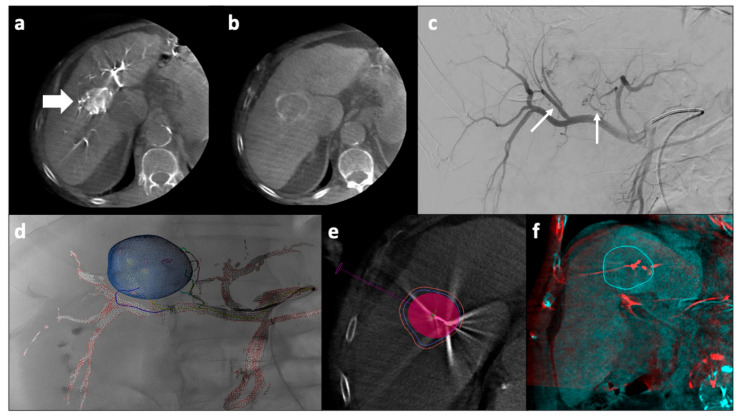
Percutaneous microwave ablation and trans-arterial chemoembolization for a large HCC nodule. For treating large single HCC nodules, a combination of ablation and embolization may be used. After arterial femoral access and catheterization of the celiac trunk and hepatic artery, a CBCT is performed that confirms the presence of a large nodule with arterial wash-in (a, arrow) and venous wash-out (b), consistent with HCC. The angiographic study demonstrated the presence of two main arterial feeders (thin arrows, c). Dedicated software can be used to delineate the target vessels and to plan the endovascular procedure (d). A dedicated software is also available to predict the ablation volume which is virtually created on a CBCT performed after having positioned the antenna inside the tumor, before starting the ablation (e,f). CBCT: cone-beam computed tomography; HCC: hepatocellular carcinoma.
